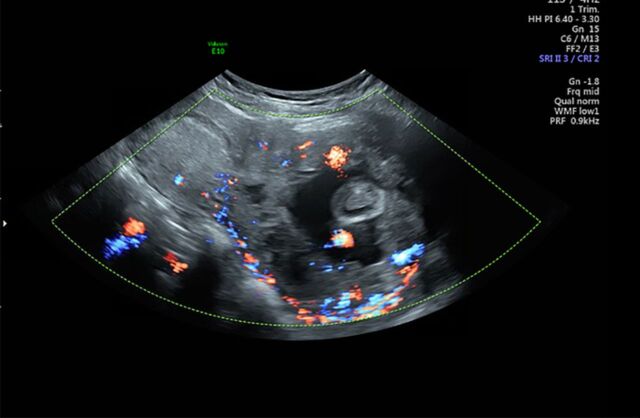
Ultrasound with Doppler flow demonstrating the vascularity surrounding the cervical ectopic pregnancy.
